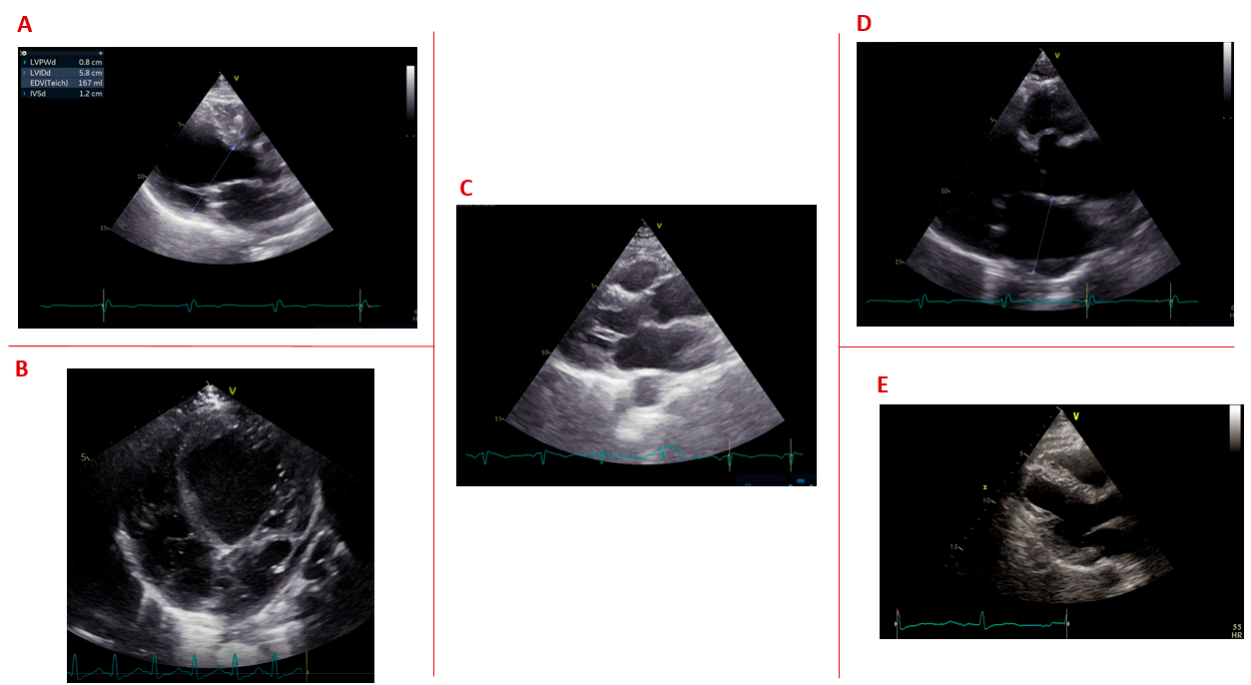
Overview of the echocardiographic structural abnormalities in DM1 patients. (A) Left ventricular dilatation; (B) myocardial non-compaction; (C) mitral valve prolapse; (D) left atrial enlargement; (E) left ventricular hypertrophy.
